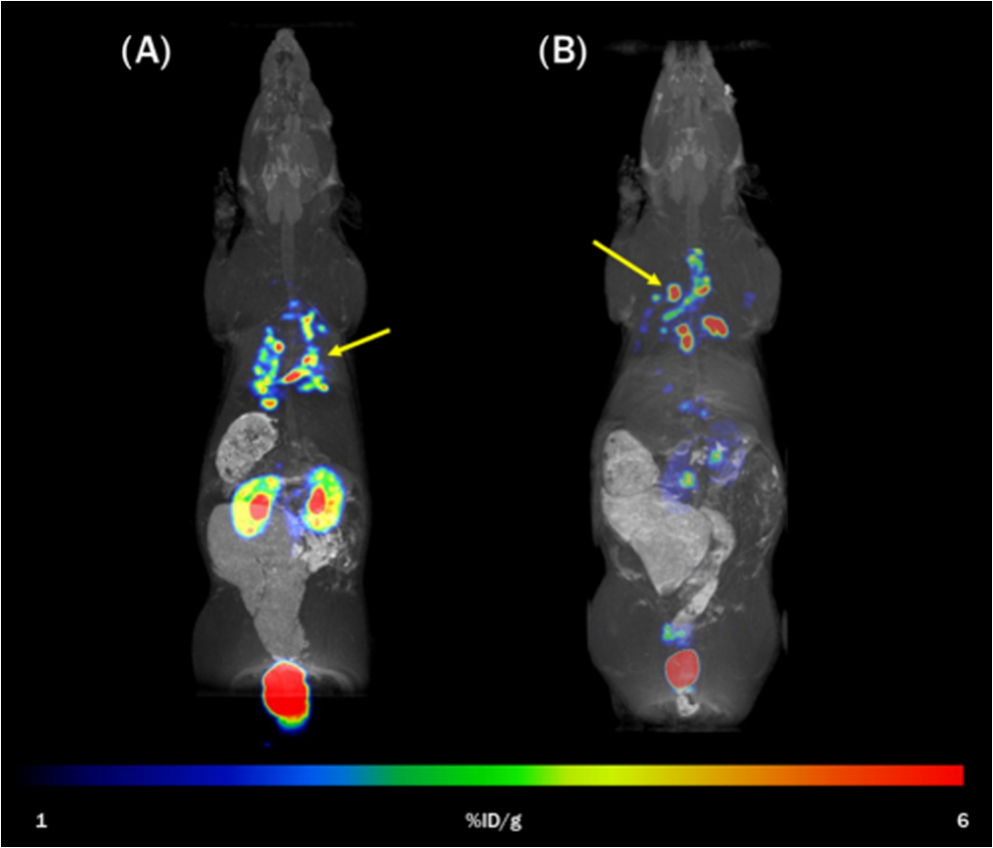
Static PET/MRI MIP images of [^68^Ga]Ga(III)-FOX 2–5
